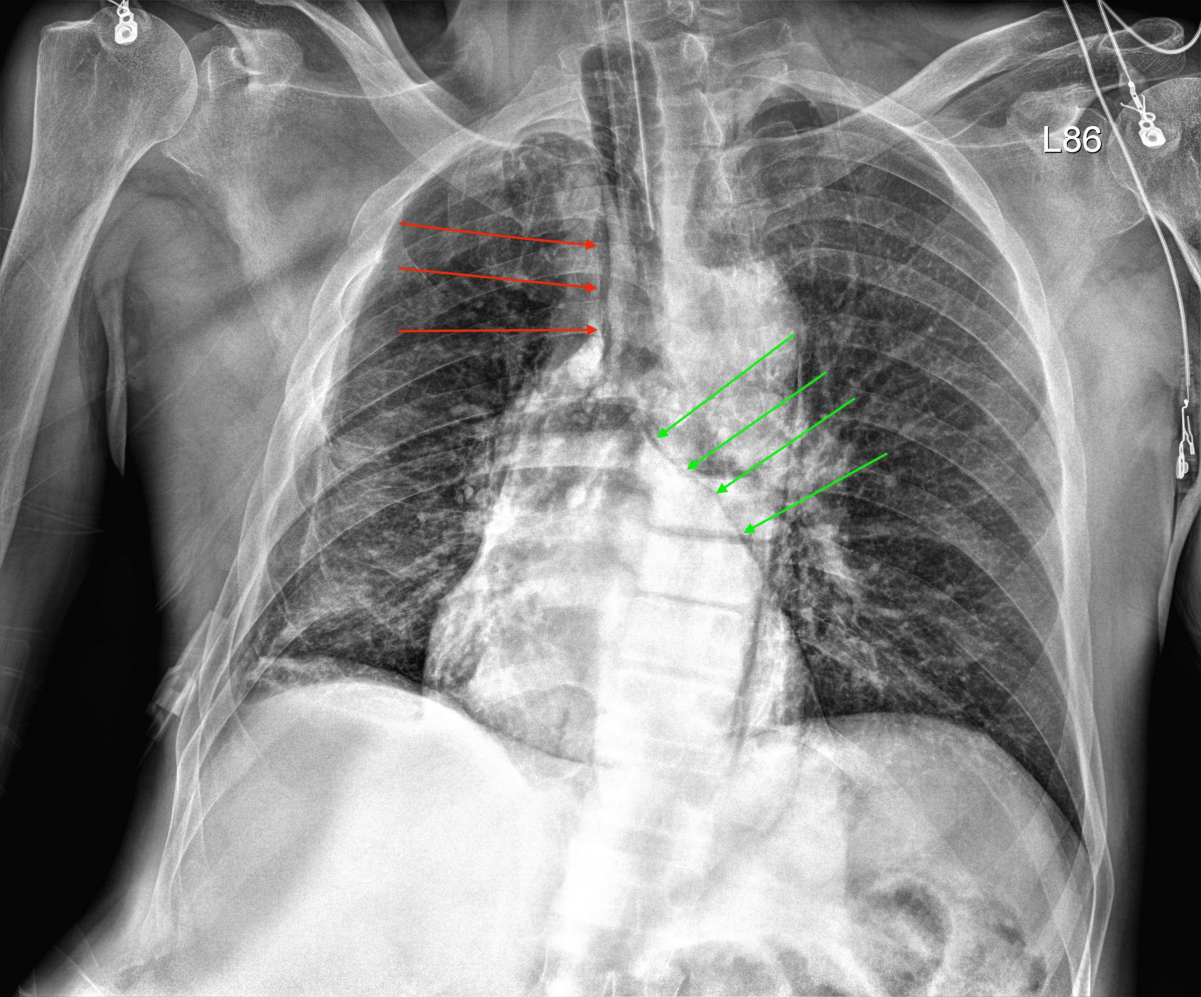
Chest X-ray taken in the operating room Red arrows show pneumomediastinum, and green arrows show pneumopericardium
